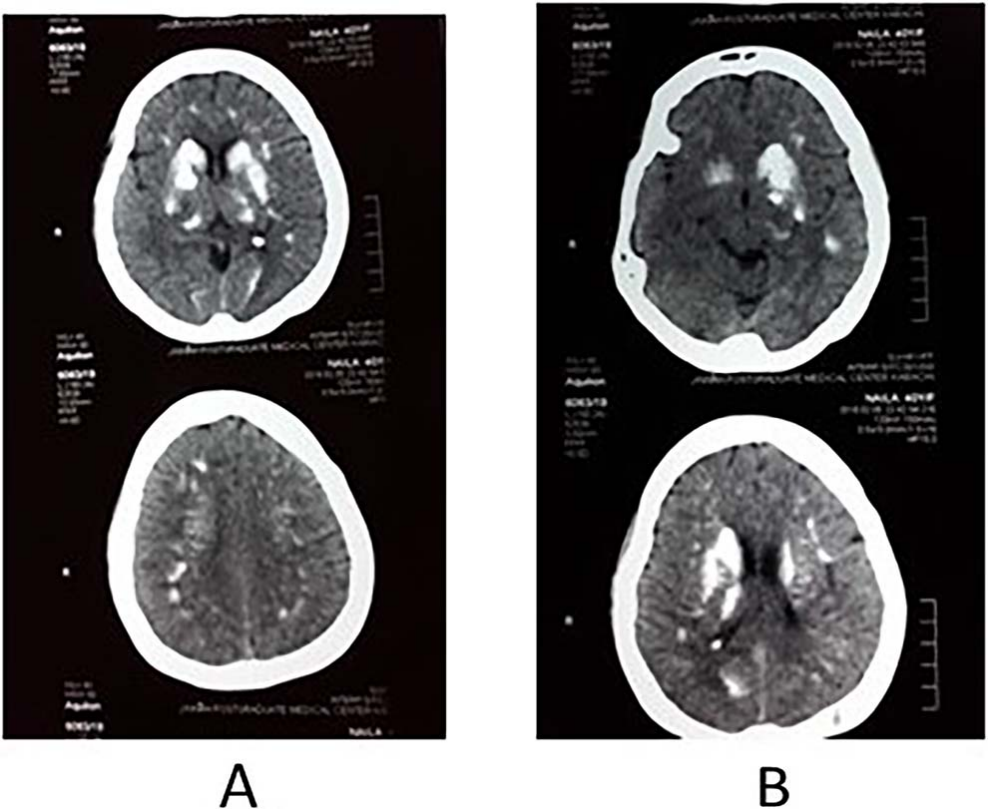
Noncontrast-enhanced computed tomography scan of the (A) left and (B) right cerebral hemispheres showing calcifications in the basal ganglia and thalami.
